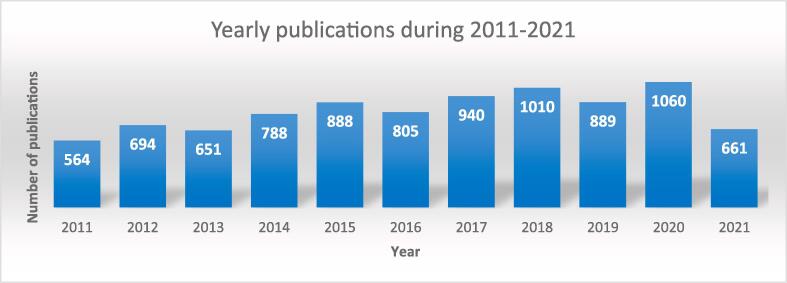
Google Scholar research on publications containing “sonochemistry” “sonochemical” or “ultrasound-assisted” words.
